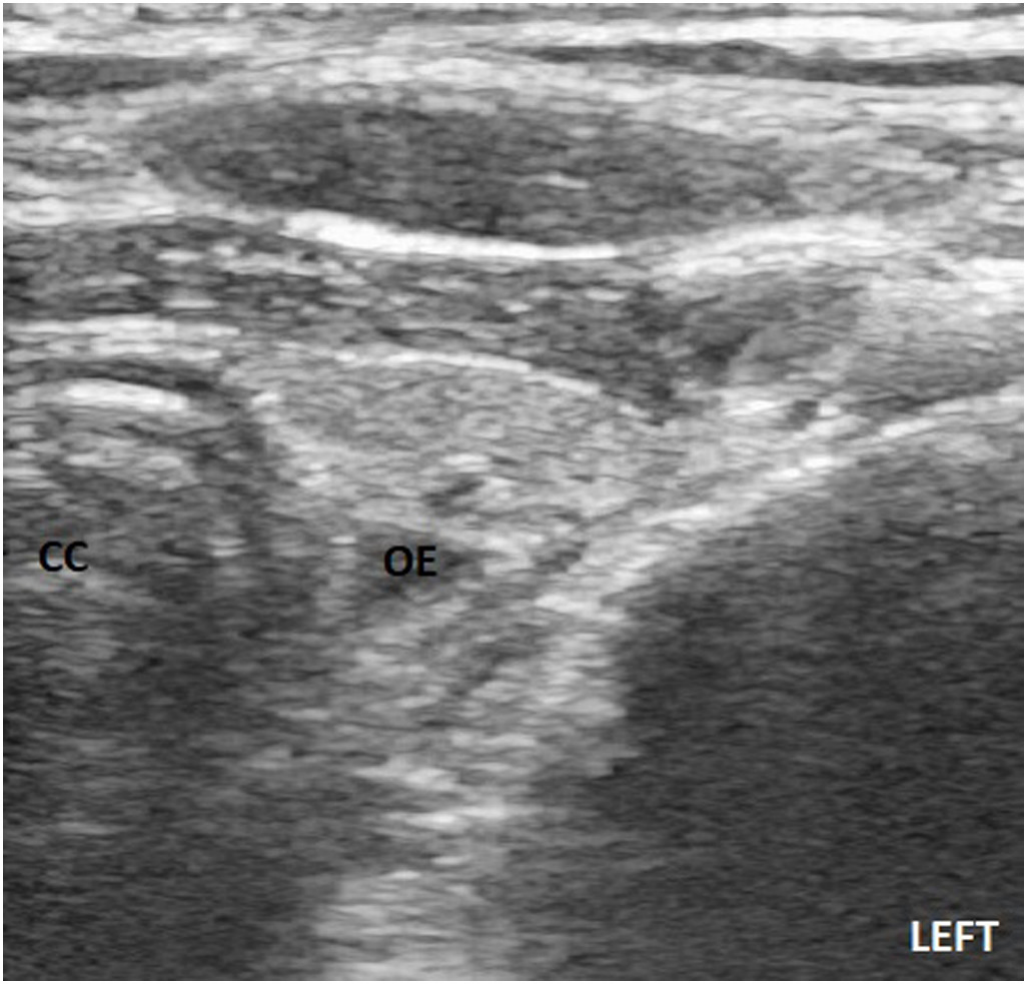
Ultrasound image of oesophagus after paralaryngeal pressure.
